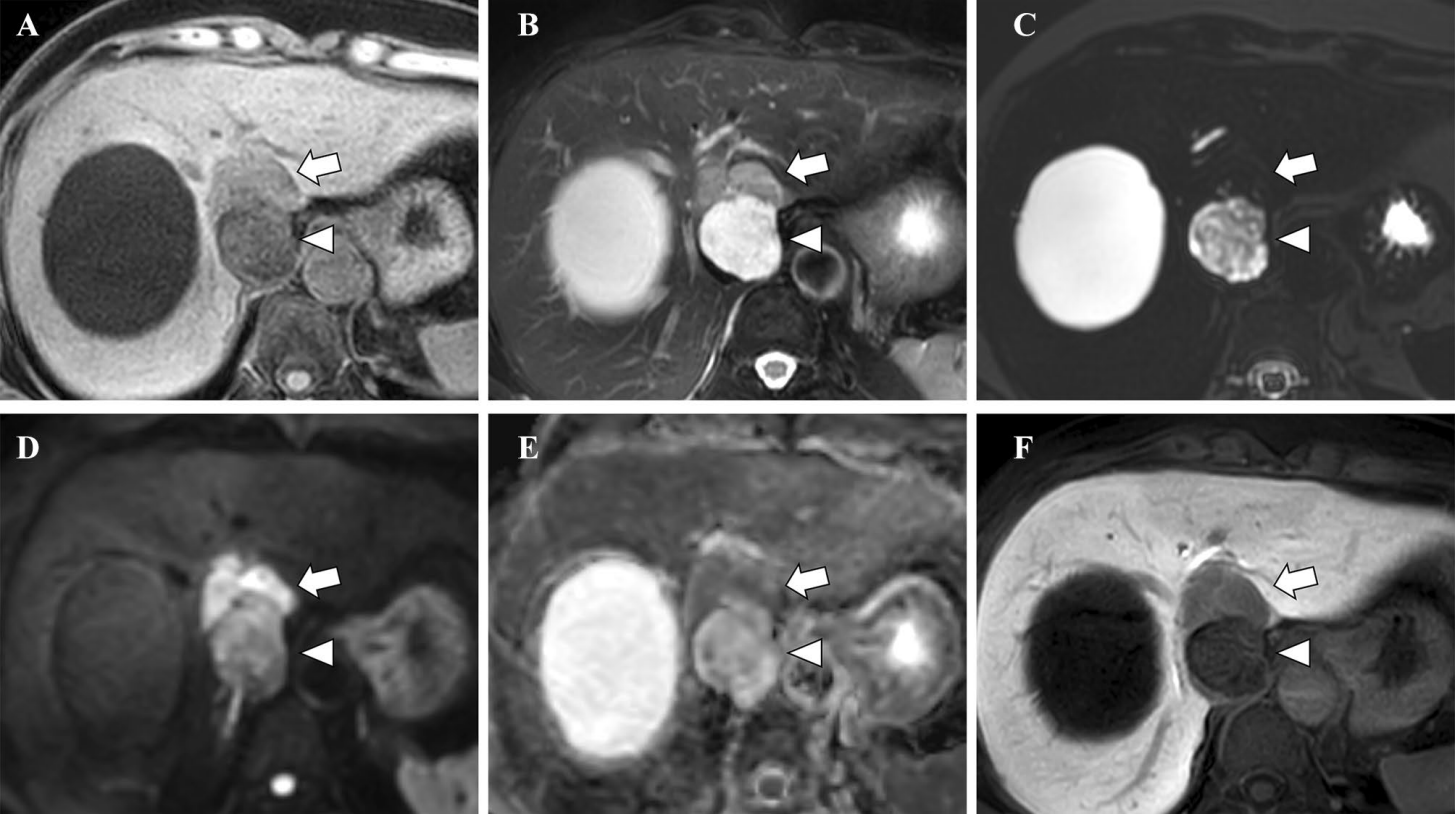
On gadoxetic acid-enhanced MR imaging, the ventral portion exhibited slightly low signal intensity on T1-weighted image (A arrow), slightly high signal intensity on fat-saturated T2-weighted image (B arrow), markedly low signal intensity on heavily T2-weighted image (C arrow), diffusion restriction (D diffusion-weighted image [b = 800]; E apparent diffusion coefficient [ADC] map, arrow, ADC = 1.1 × 10^−3^ mm^2^/s), and no uptake of gadoxetic acid in the hepatobiliary phase (F arrow). The dorsal portion showed low signal intensity on T1-weighted image (A arrowhead), high signal intensity on fat-saturated T2-weighted (B arrowhead) and heavily T2-weighted image (B arrowhead), no diffusion restriction (D diffusion-weighted image [b = 800]; E ADC map, red arrow, ADC = 1.5–2.5 × 10^−3^ mm^2^/s), and no uptake of gadoxetic acid in the hepatobiliary phase (F arrowhead)
